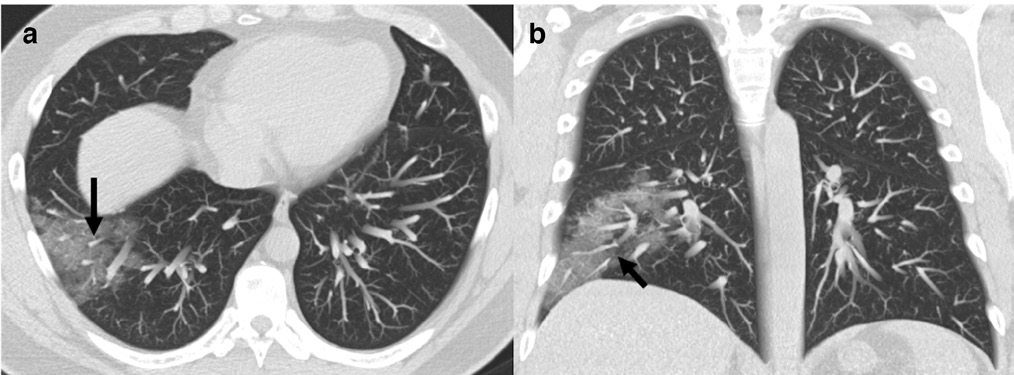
Axial (A) and Coronal (B) (MIP CT images of a patient with COVID-19 infection showing peripheral ground-glass opacity with focal vascular enlargement (black arrows) within the diseased lung. MIP, maximum intensity projection.
